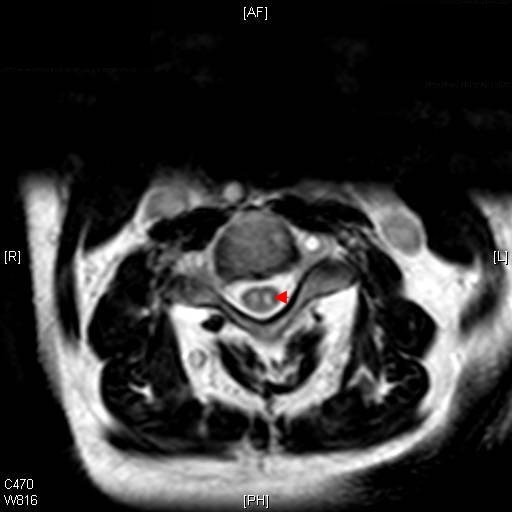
Sagittal T2-weighted MRI scan showing an abnormally high signal of the spinal cord extending from C3 down to the lower end plate of C5.
